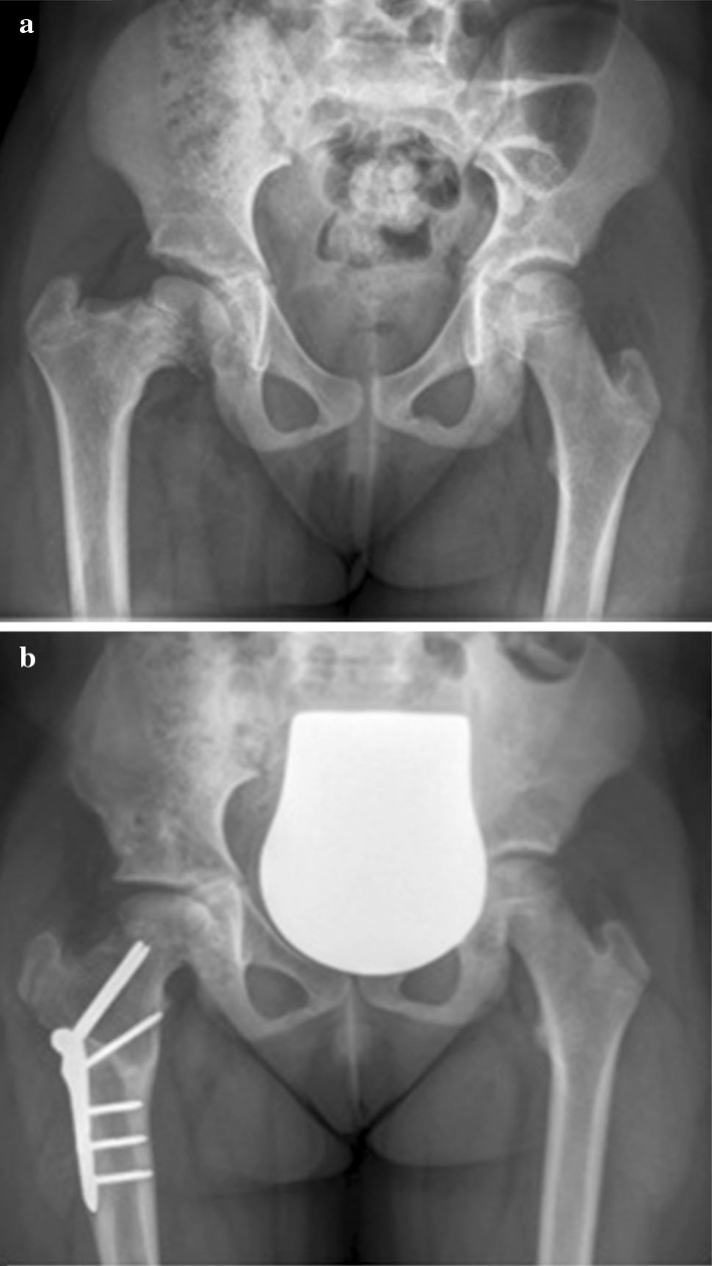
a Preoperative X-ray of a 10.5-year old girl presenting with congenital femoral defect. b Good reduction is achieved after femoral valgisation osteotomy (30°), and at 1.2 years of follow-up, reduction is maintained with good head coverage
